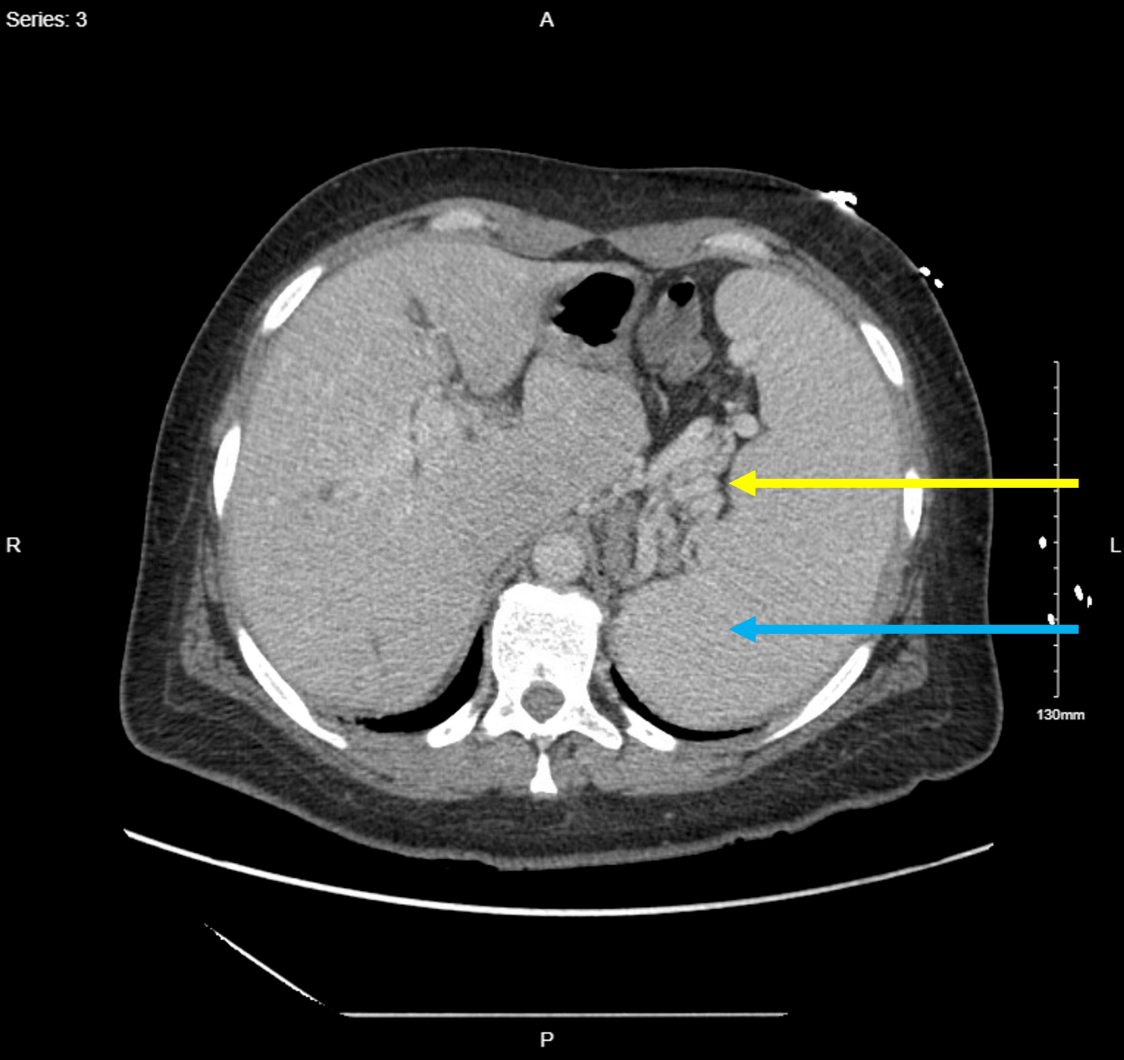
Computed tomography scan of the abdomen and pelvis with intravenous contrast, axial view demonstrating perisplenic veins (yellow arrow) and splenomegaly (blue arrow).
